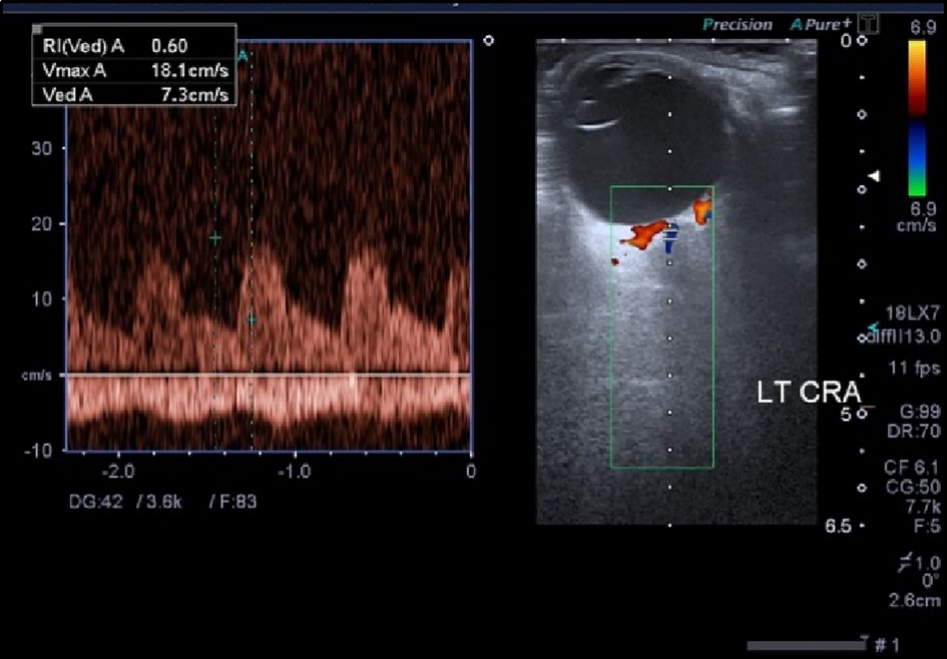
CDI of the left eye of a diabetic patient showing the Doppler spectral waveform of CRA and CRV. The flow velocity waveform in the CRA is of low amplitude and typical peaks for systolic and diastolic velocities are seen above the zero line. The flow velocity waveform in the CRV (almost monophasic) presents below the zero line.
